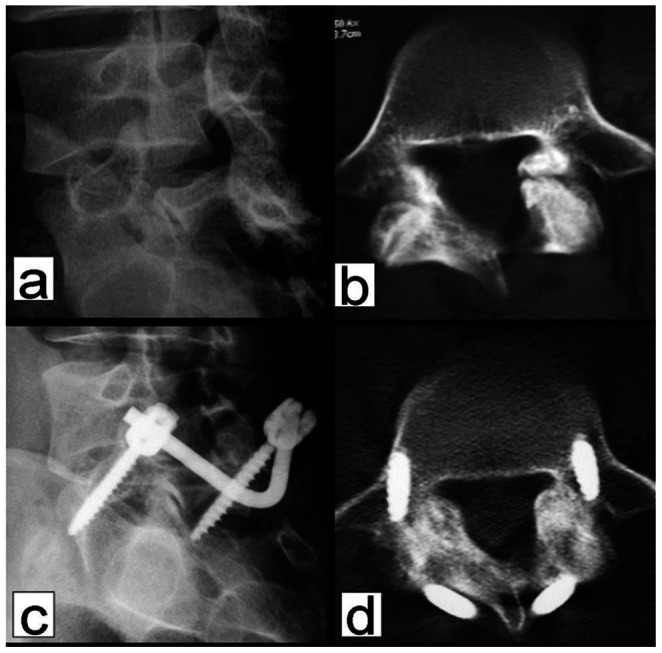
A 21-year old male patient with isthmic spondylolysis for 7 months failed conservative therapy. Preoperative oblique x-ray and CT scan using bone windows reveal isthmic spondylolysis at L5 (a and b). At 6 months postoperatively, the oblique view shows an indistinct interface between the isthmus and bone graft with increased density (c). CT scan on bone windows shows healing of the isthmic bone graft (d).
